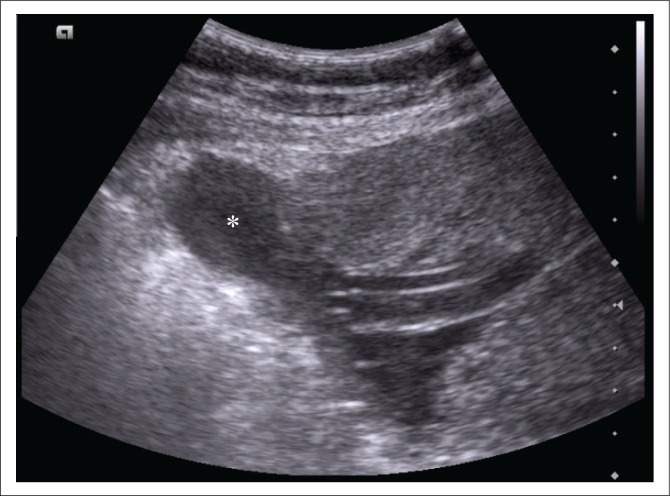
Transverse abdominal ultrasound demonstrating a well-circumscribed, anechoic structure (asterisk), adjacent to placental tissue, occupying the uterine fundus on the right. Communication with the uterine cavity is evident, in keeping with the amniocele demonstrated on magnetic resonance imaging.
